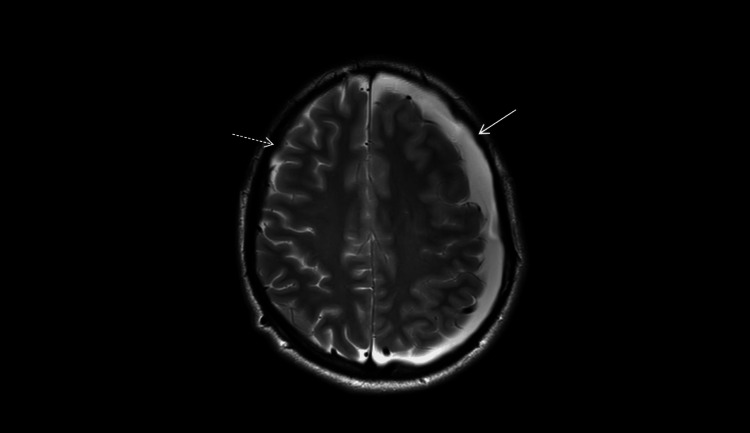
MRI (March 5, 2025; axial T2-weighted; 4-mm slices; 0.5-mm gap). Complete resolution of the right convexity subdural hygroma (dashed arrow) with persistence and interval enlargement on the left (solid arrow), compared with the early postoperative study (Figure 4).
